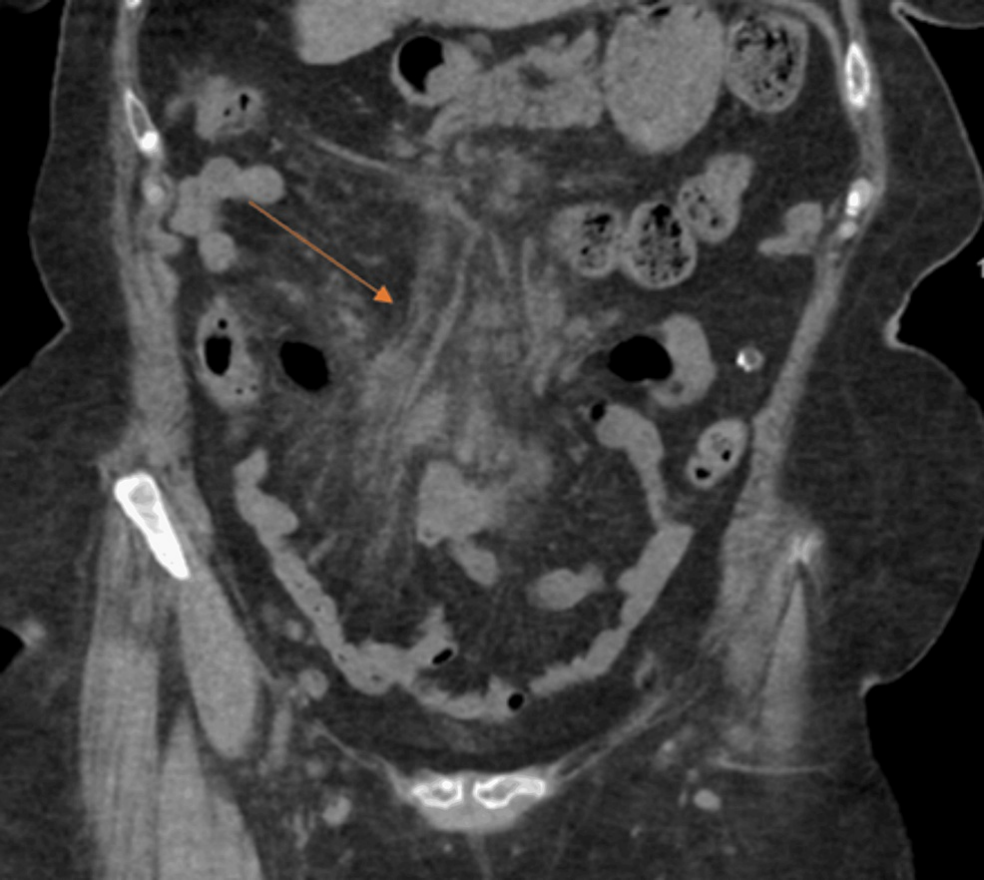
CT of the abdomen/pelvis with contrast. Fat stranding visualized (orange arrow).
